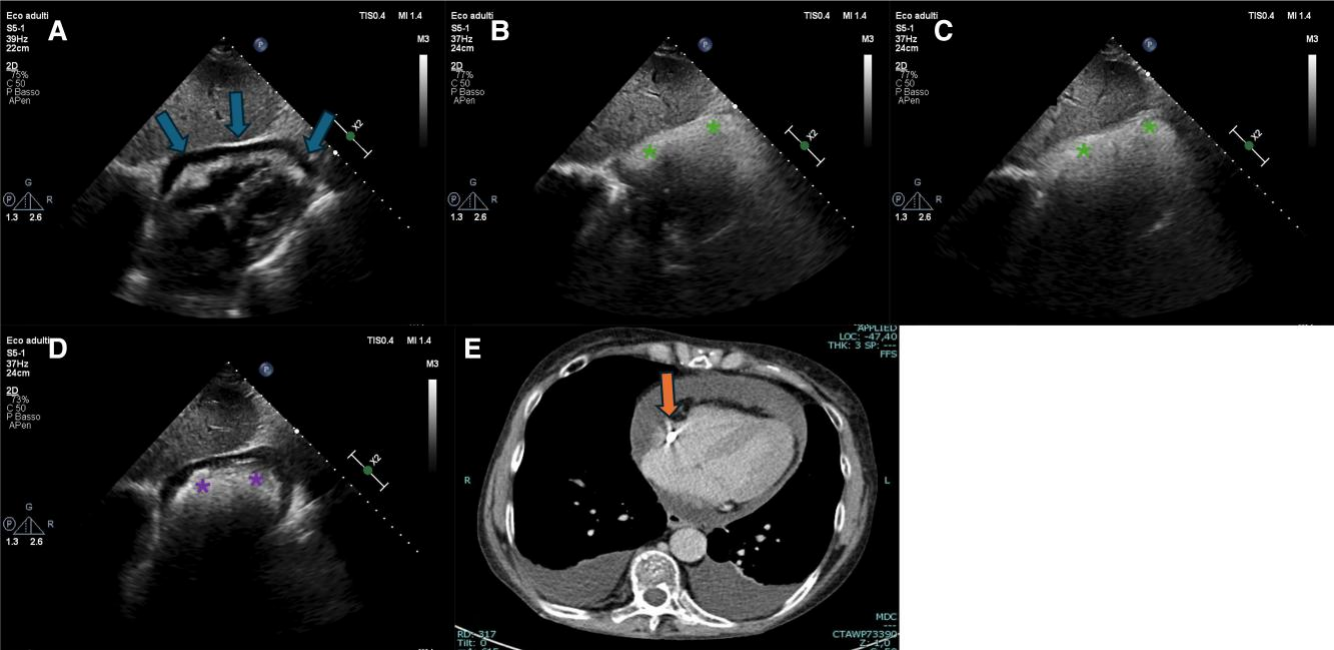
(*A*) Subcostal view showing circumferential pericardial effusion (arrow annotations). (*B*, *C*) Subcostal view showing bubbles in pericardial cavity (star symbols) after contrast injection from the middle and the distal lumens respectively (see Supplementary material online, *Videos S1* and *S2*). (*D*) Subcostal view showing bubbles in right chambers (star symbols) after contrast injection from the proximal lumen (see Supplementary material online, *Video S3*). (*E*) Computed tomography scan showing the catheter tip’s location against the right atrial wall (arrow annotation).
